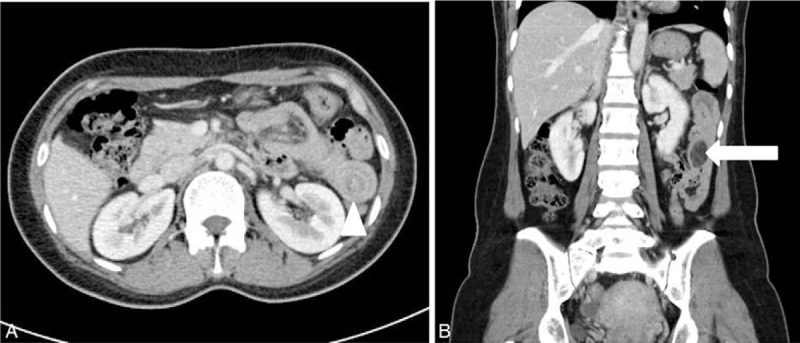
An axial abdominal contrast-enhanced computed tomography (CT) image shows dilated loop of small bowel consistent with a possible small bowel obstruction and a potential target sign (white arrow head) in the left upper quadrant (A). A coronal CT image showing a well-defined, low-fat-density mass (white arrow) (B), which we assumed was the lead point for the intussusception.
